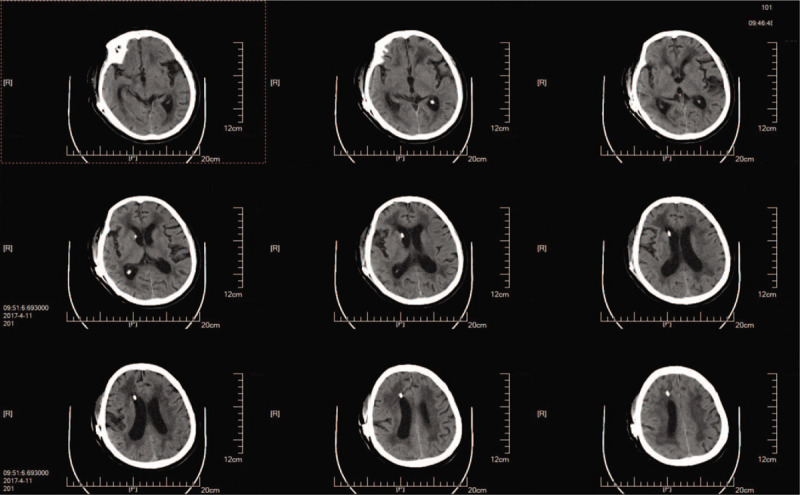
Postoperative head computed tomography scan obtained during first admission showed the relief of ventricular enlargement and paraventricular edema.
